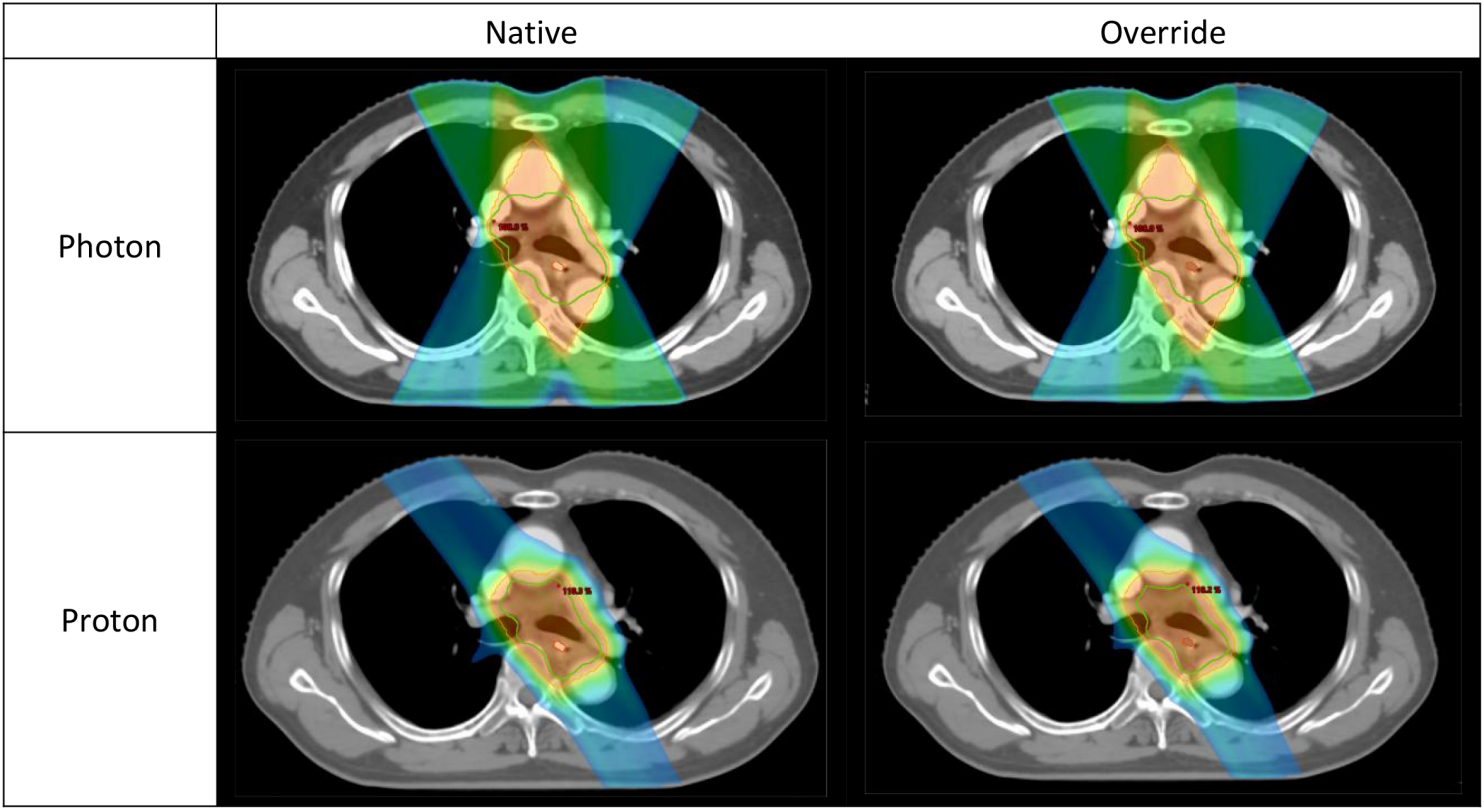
Representative dose distributions in photon and proton radiotherapy. Representative axial dose distributions for the same patient comparing photon and proton radiotherapy plans calculated on native CT images and with titanium clip density override. The upper row shows photon plans and the lower row shows proton plans; the left column represents native plans and the right column represents override plans. The orange region denotes the 95% isodose, the green contour indicates the PTV in photon plans and the CTV in proton plans, and the red contour marks the endoscopic titanium clip. The dose distributions visually support the quantitative results in Tables 2 and 3.
